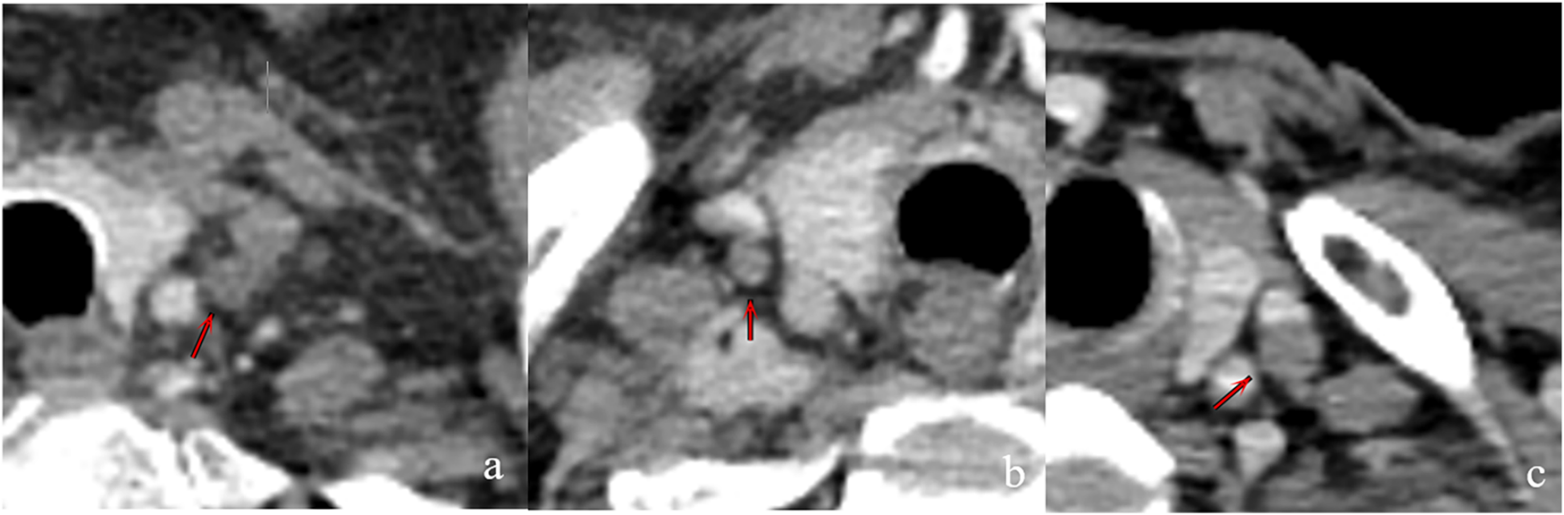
A 72-year-old male with ESCC. a Enhanced CT scan shows a small left SCLN with SAD of 5.8 mm (red arrow), exhibiting a heterogeneous configuration. Subsequent US-FNAB of the SCLN revealed negative pathological results. b A 73-year-old male with ESCC. Enhanced CT scan shows a small right SCLN with SAD of 4.7 mm, exhibiting a heterogeneous configuration. Subsequent US-FNAB of the SCLN revealed negative pathological results. c A 66-year-old male with ESCC. Enhanced CT scan shows a small left SCLN with SAD of 8.8 mm, exhibiting a heterogeneous configuration. Subsequent US-FNAB of the SCLN revealed positive pathological result
